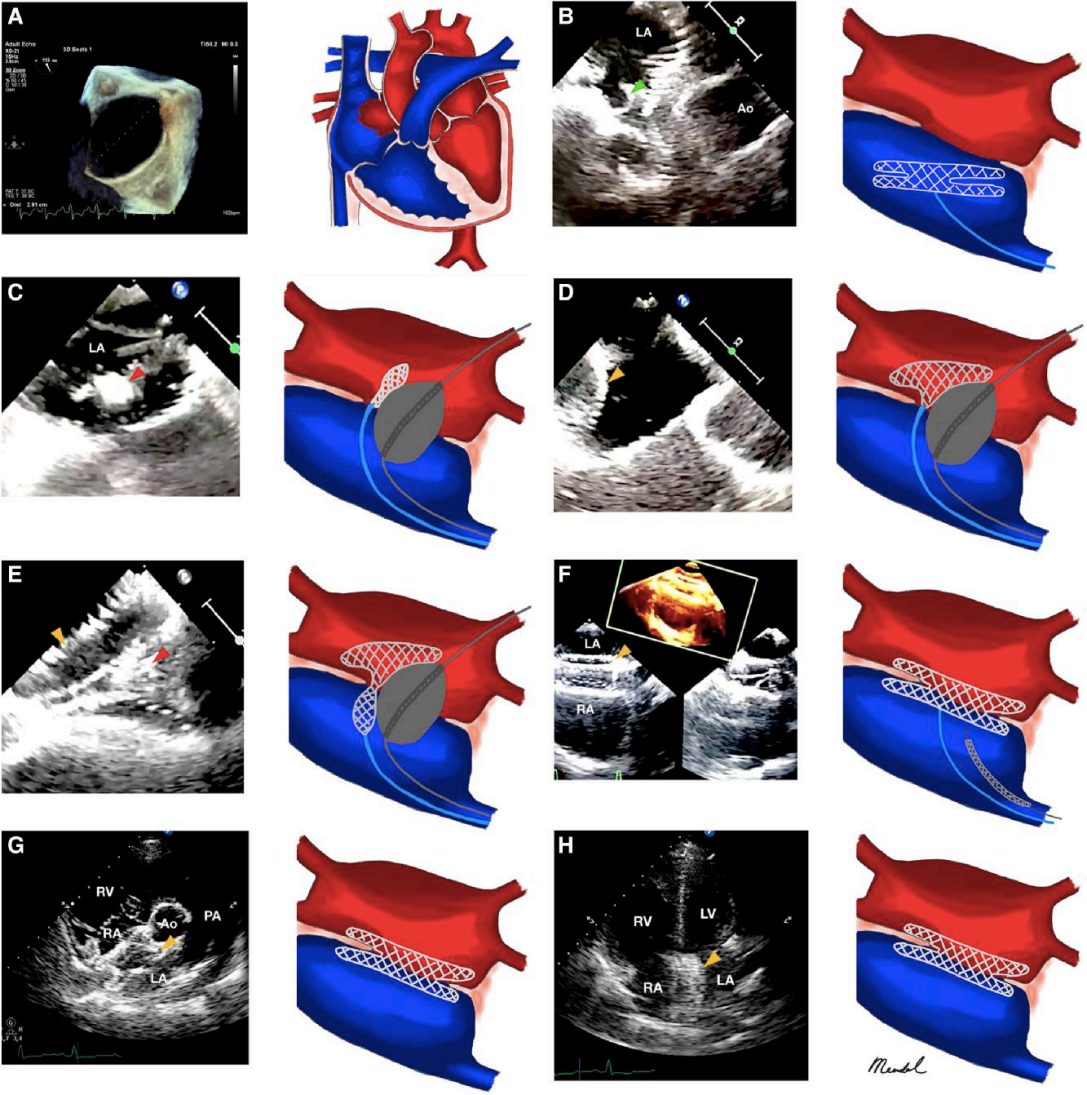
Balloon-assisted non-fluoroscopy secundum atrial septal defect closure in pregnant women. (*A*) An oval-shaped 22 × 33 mm-sized secundum atrial septal defect with a left-to-right (L-to-R) shunt and transvalvular gradient of the tricuspid valve of 55 mmHg. (*B*) A transoesophageal echocardiography short-axis image indicates that the left atrial disc dislodged from the right atrium and could not engage correctly (*green arrowhead*). (*C*–*E*) A balloon-assisted approach using a sizing balloon of No. 34 mm (*red arrowhead*). After the device was finally stowed, a wiggle test was conducted to make sure that it remained stable (*orange arrowhead*), which was confirmed in a transoesophageal echocardiography bicaval view, (*F*) transthoracic echocardiography parasternal long-axis view (*G*), and apical four-chambered view (*H*).
